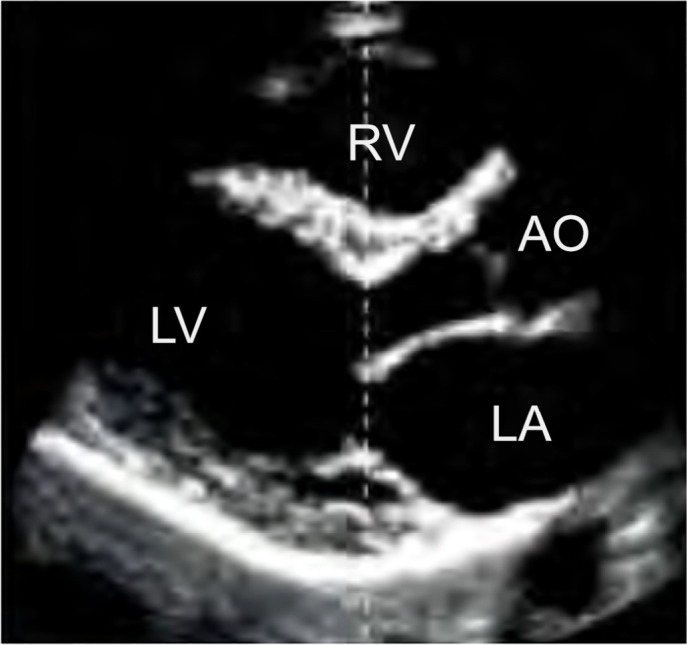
Parasternal longitudinal window.
